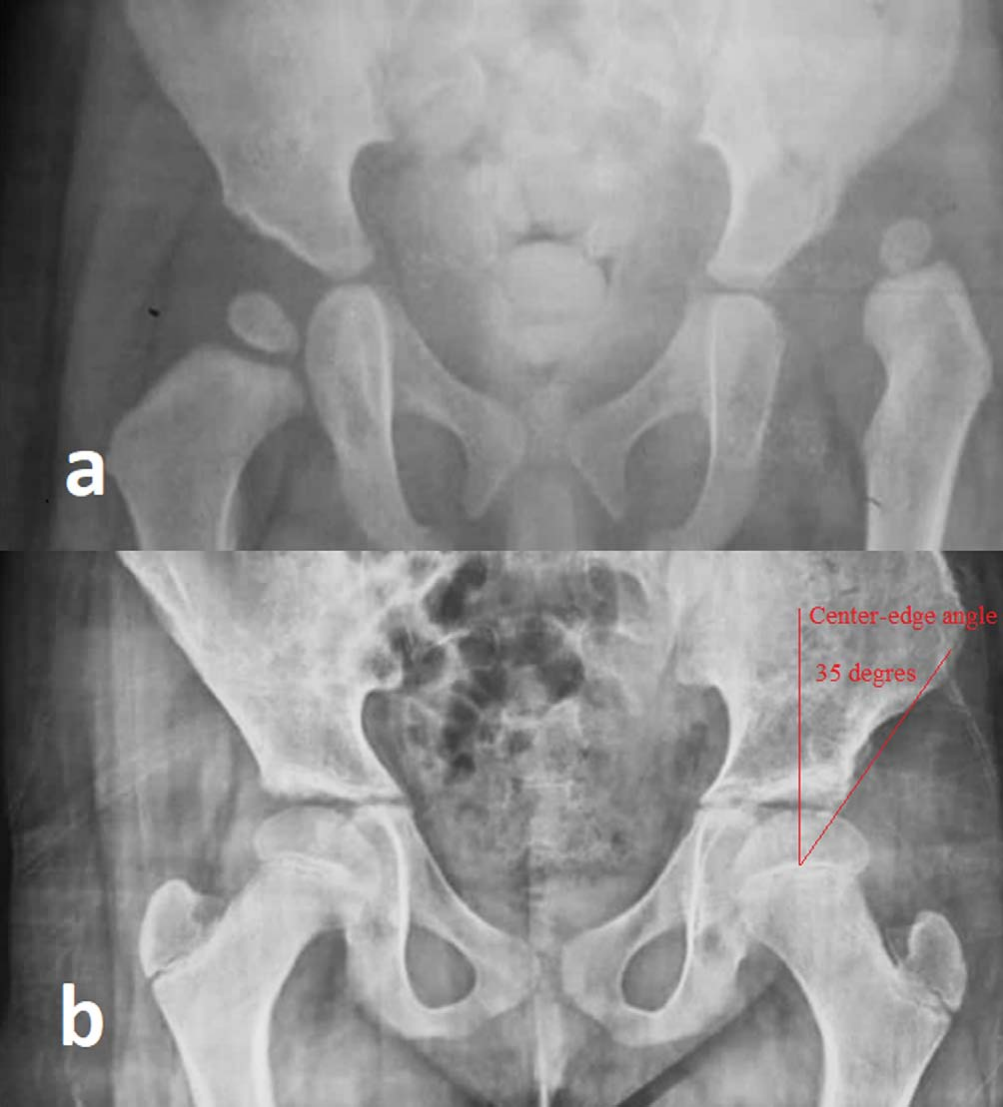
(a) Preoperative radiograph of a girl 1.5 years old of age with right DDH; (b) radiograph at the age of 8 with center-edge angle(CEA) of 35° (Severin score at I) at 6.5 years follow-up after open reduction.
